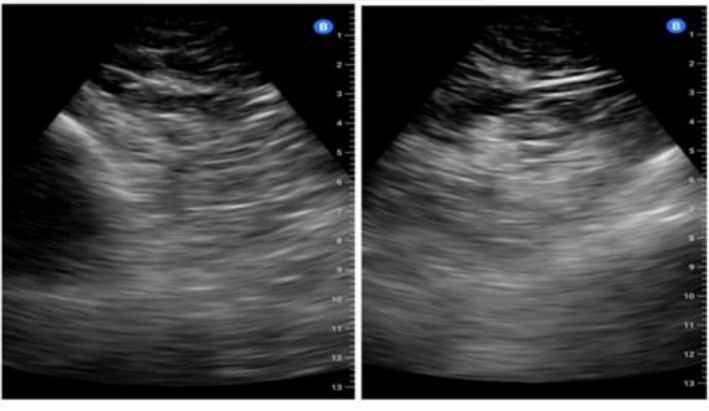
Patient 3. Ultrasound of the left and right lung bases, respectively, in posterior view, showing small foci of fluid bronchograms scattered throughout the parenchyma, slightly more organized in the right lung.
